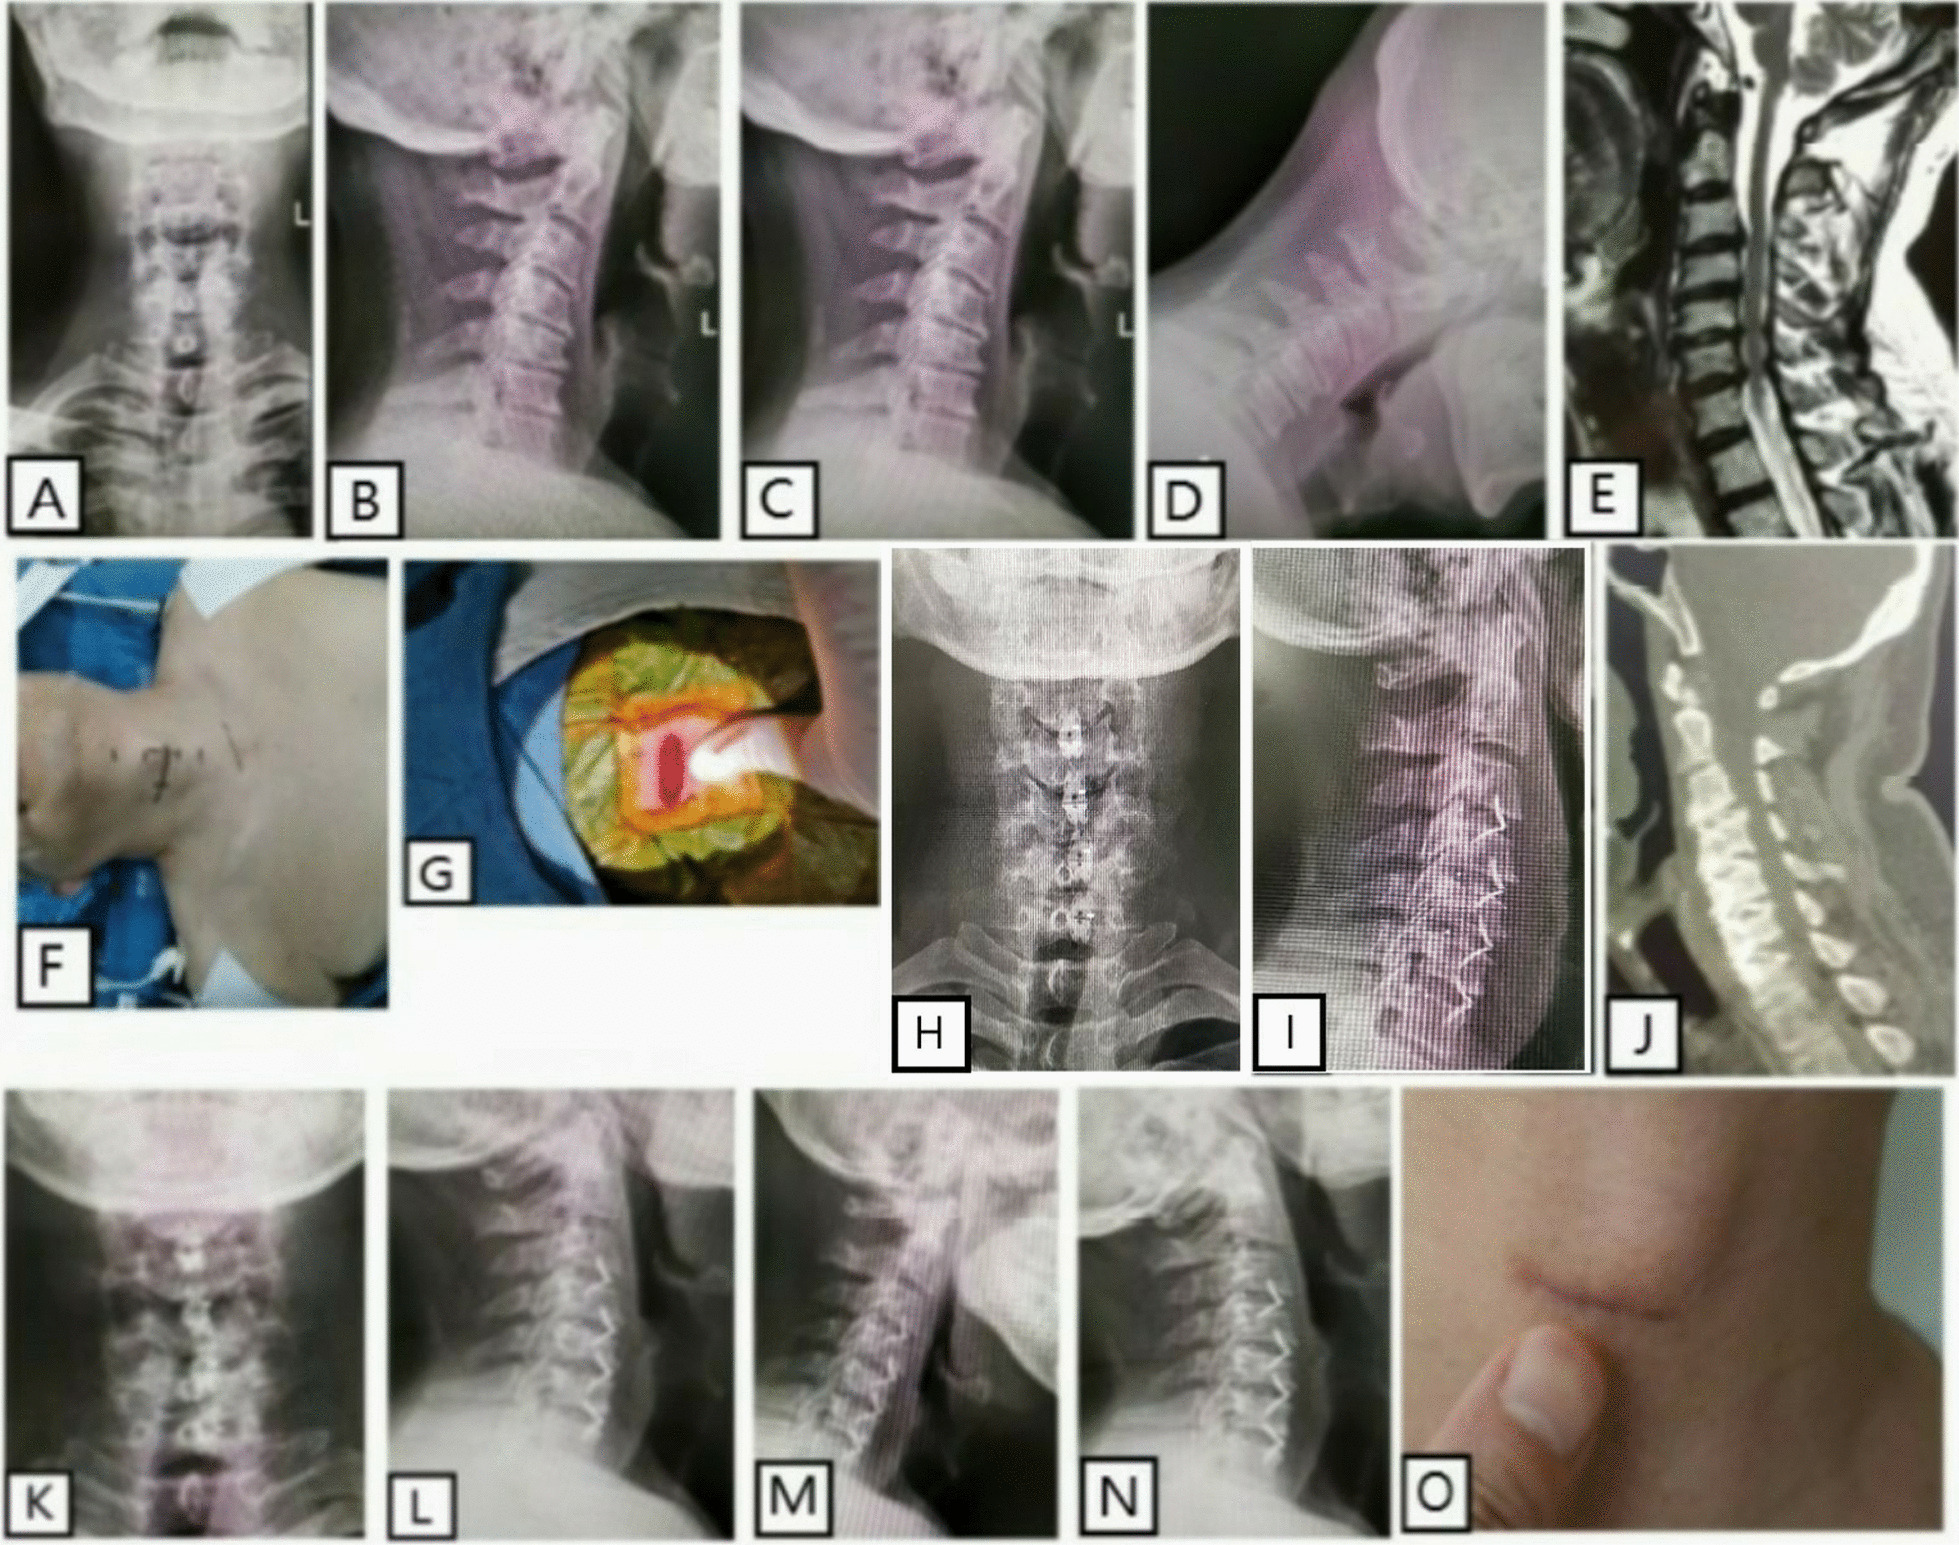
ACDF treatment for multisegments CS. A preoperative anteroposterior radiograph. B Preoperative lateral radiograph. C–D Preoperative hyperextension and flexion radiograph. E Preoperative cervical MR. F Preoperative incision. G Intraoperative incision. H Postoperative anteroposterior radiograph. I Postoperative lateral radiograph. J–L Postoperative CT and X-ray at the follow-up. M–N Postoperative hyperextension and flexion radiograph, and O postoperative incision
